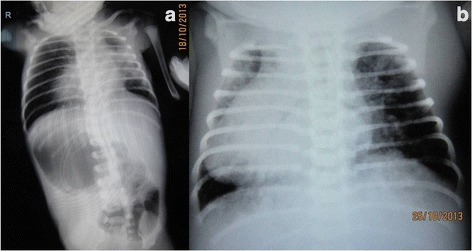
Plain roentgenograms of her chest and abdomen revealing dextrocardia, liver opacity on the left side of her abdomen, and a stomach bubble on the right side suggestive of situs inversus. Few dilated loops in upper abdomen with absence of distal air (a). A postoperative X-ray showing a dilated cardiac shadow on the right side along with infiltration of the lung fields (b)
